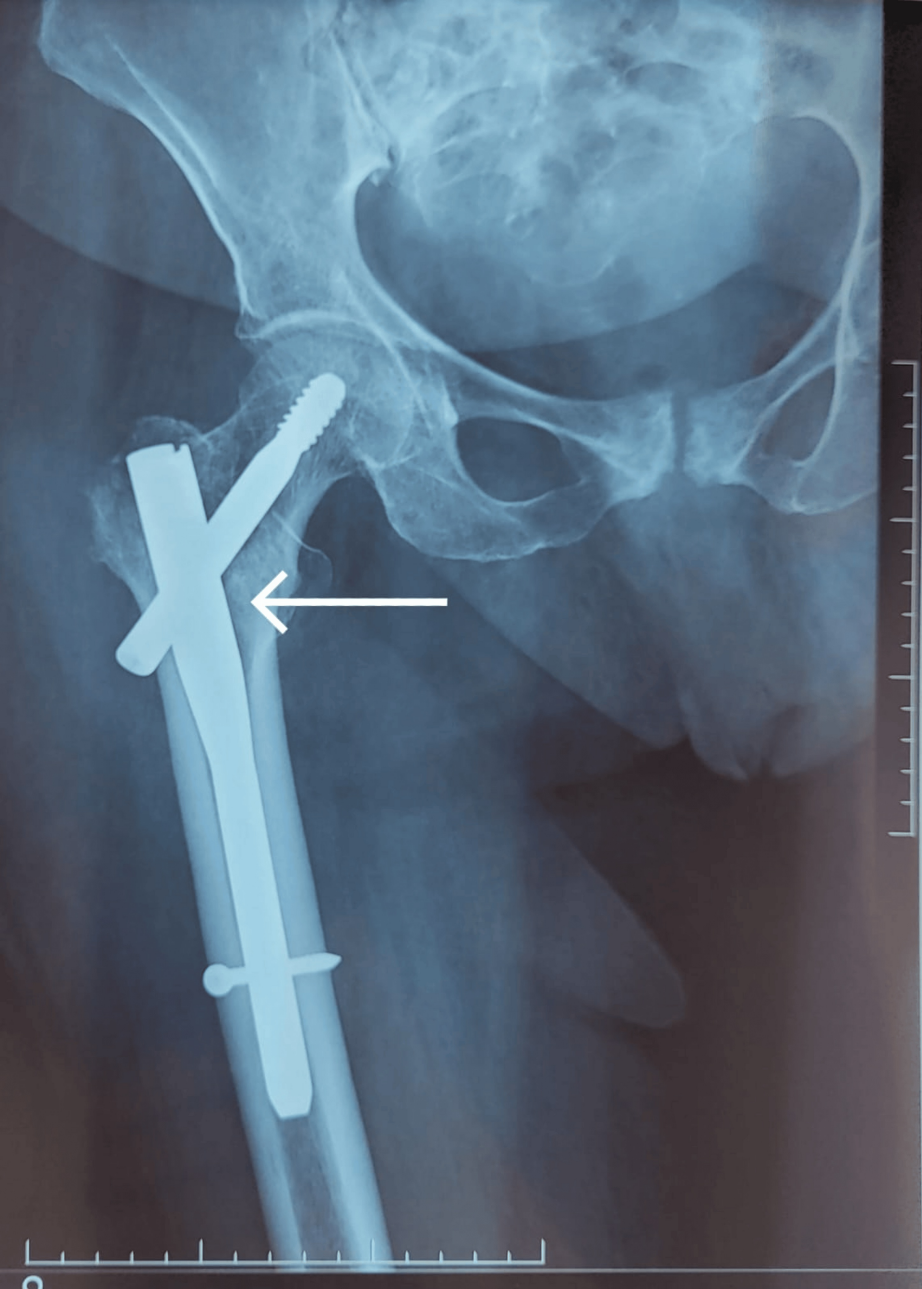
Postoperative radiograph showing the gamma nail (arrow)
